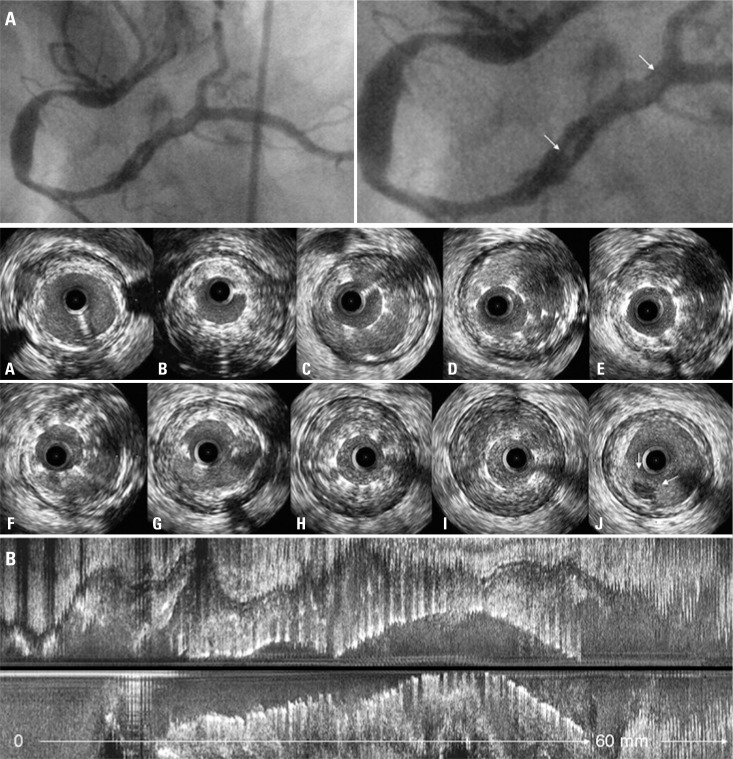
Diagnostic intravascular ultrasound was performed to assess the angiographic filling defect at the right coronary artery (A, white arrow in the angiography). The intravascular ultrasound images are shown from proximal (A) to distal (J) in figure B. There was severe malapposition and underexpansion throughout the entire length of the stents. Notice the space between the stent strut and the intima and the blood speckle/thrombus behind the stent struts in the axial (B through I) as well as the longitudinal view (at the bottom). At the site of maximum stent malapposition (I), the stent area (4.99mm^2^) was smaller than lumen area (15.22mm^2^) and external elastic membrane (26.64mm^2^). The entire distal 20+mm of the stent was thrombus filled with additional thrombus on the abluminal side of the stent partly filling the area of malapposition and causing the linear filling defect on the angiogram. The small intraluminal mass on IVUS (J, small arrows) represented the tail of the thrombus with the bulk being proximal to that slice
